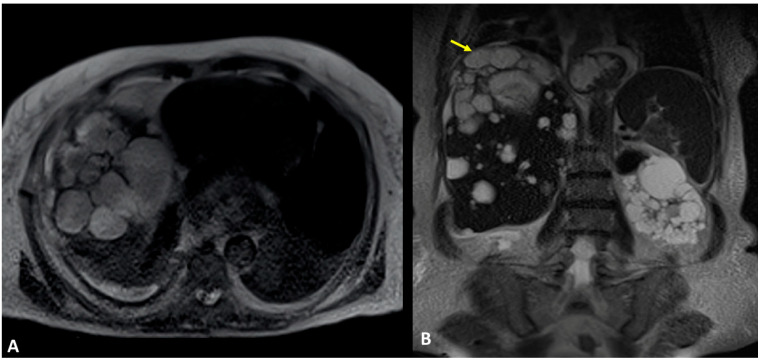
(A,B) Contrast-enhanced MRI, T2-weighted sequences, and axial (A) and coronal planes (B). In Figure (A), the right basal empyema and pleural thickening leaflets are confirmed. In Figure (B), the right lower lung lobe and a hepatic cyst (polycystosis) look strongly adjacent (arrow), without a definite fistulous tract.
